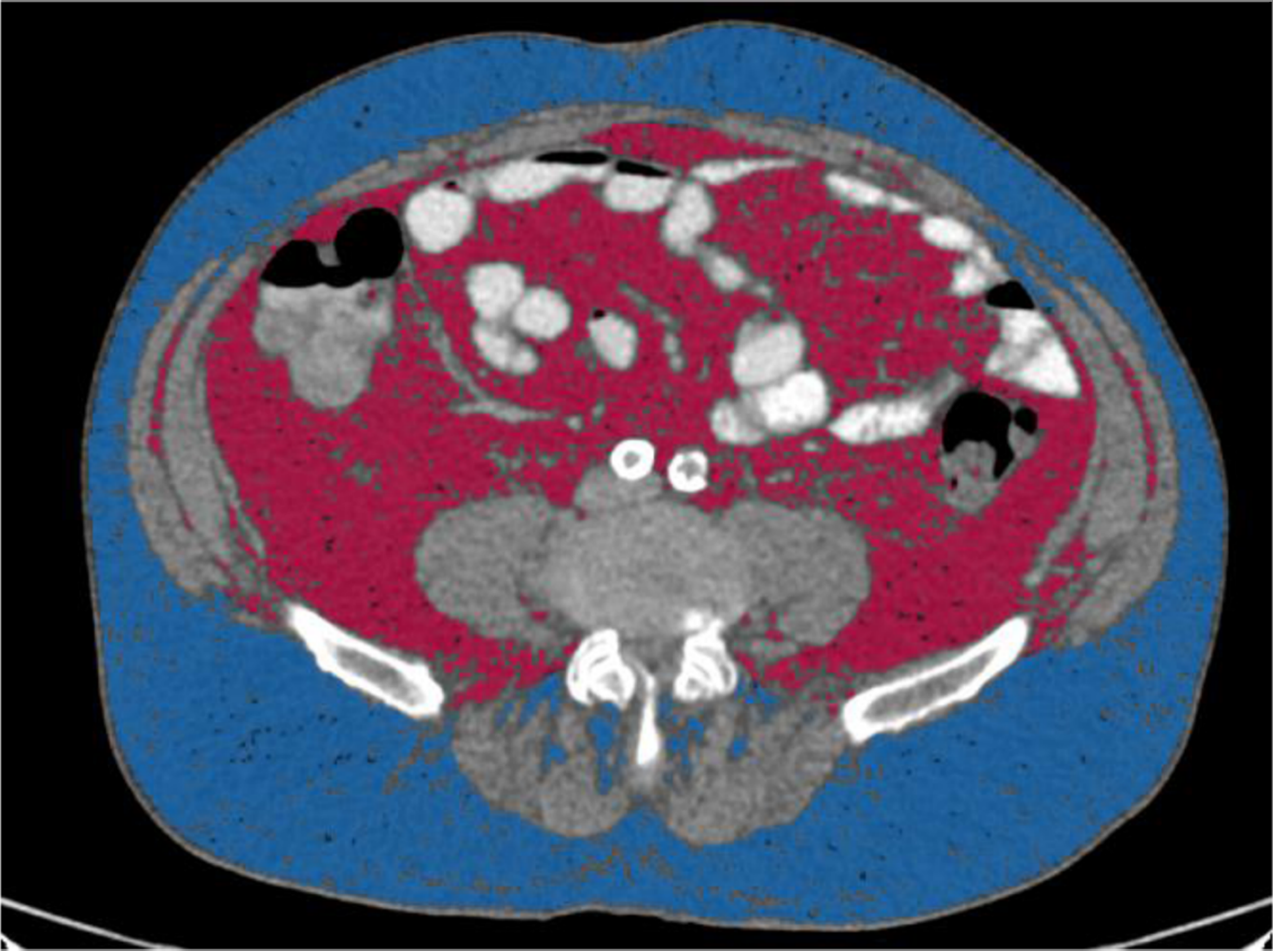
Abdominal adipose tissue segmentation. Representative CT scan obtained at the umbilicus. Subcutaneous and visceral fat are colored in blue and red, respectively.
